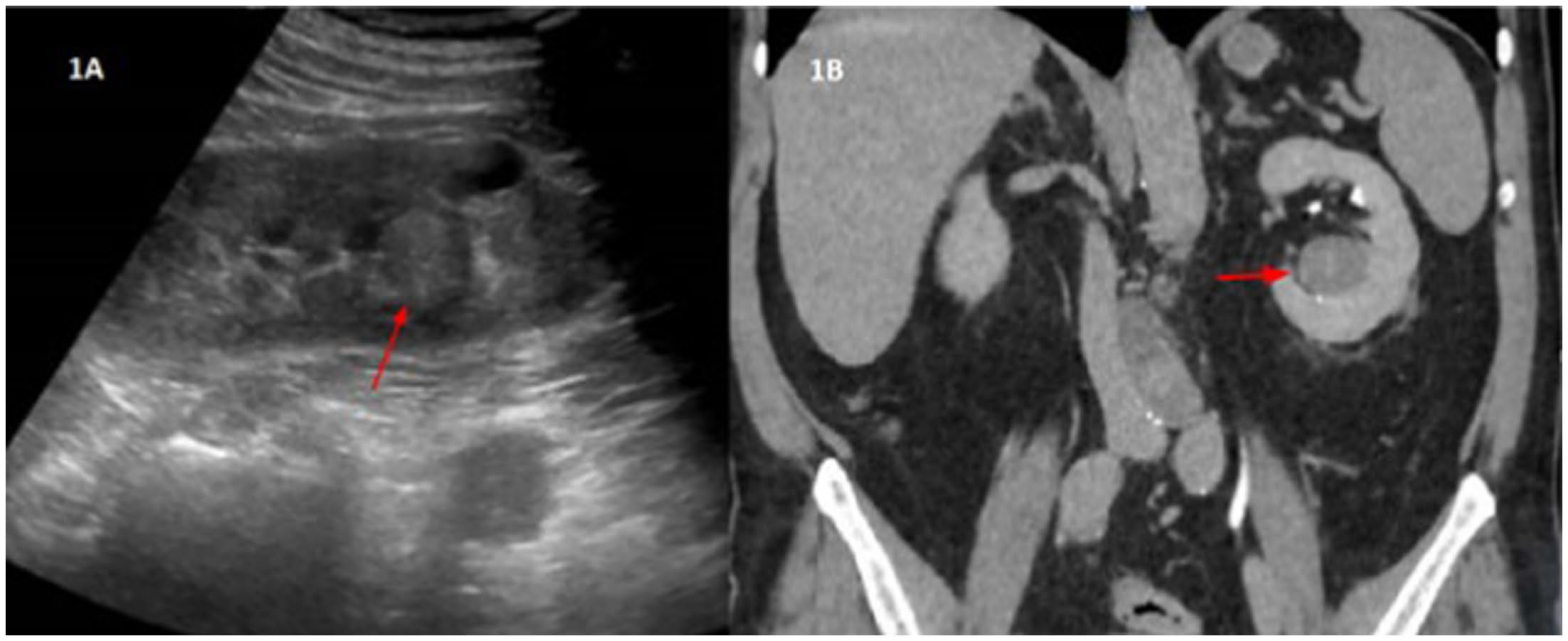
(A) Ultrasound imaging. Nodular image in the left renal sinus, of approximately 2 cm, isoechogenic with the cortex. (B) Uro-CT-Scan. In the lower portion of the left renal sinus, a nodular lesion, isodense with the renal cortex, is seen. It has homogeneous density, with rounded morphology and well-defined edges. Approximate measurements by CT are of 3.3 × 2.9 × 2.7 cm in the transverse, anteroposterior and craniocaudal axes, respectively. This lesion shows a density of 46 HU, therefore it is a solid lesion. In the portal phase it presents 56 HU, that is, it shows very slight uptake. In the excretory phase, the lesion has a density of 67 HU, which confirms a slowly progressive uptake. These findings are suggestive of papillary renal neoplasia as the first diagnostic option.
